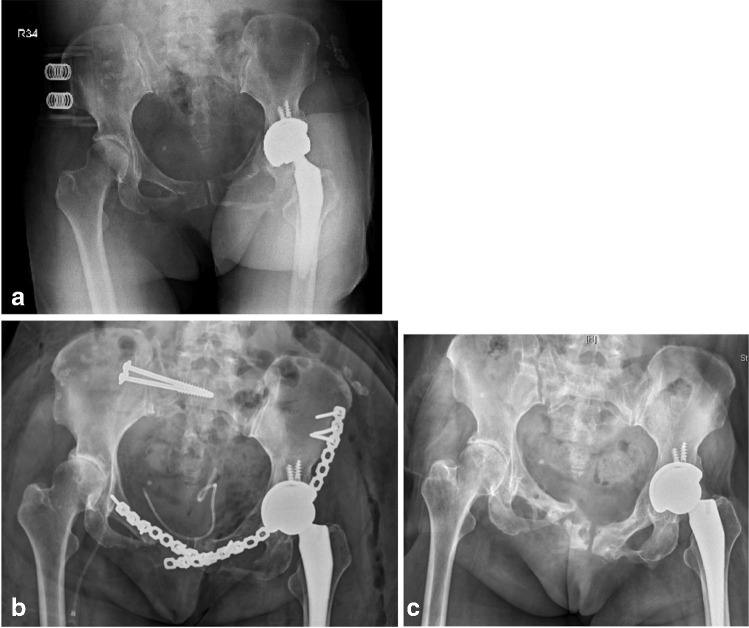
a Pre-operative AP radiographs of a 60-year-old woman with history of left THR (no. 4), showing left low anterior column fracture with right saddle type pubis fracture and sacral zone II fracture. b Post-operative AP radiograph showing anatomical reduction. c AP radiographs after removal of implants showing poor radiological outcome on right side pubic ramus but excellent radiological outcome on left hip
